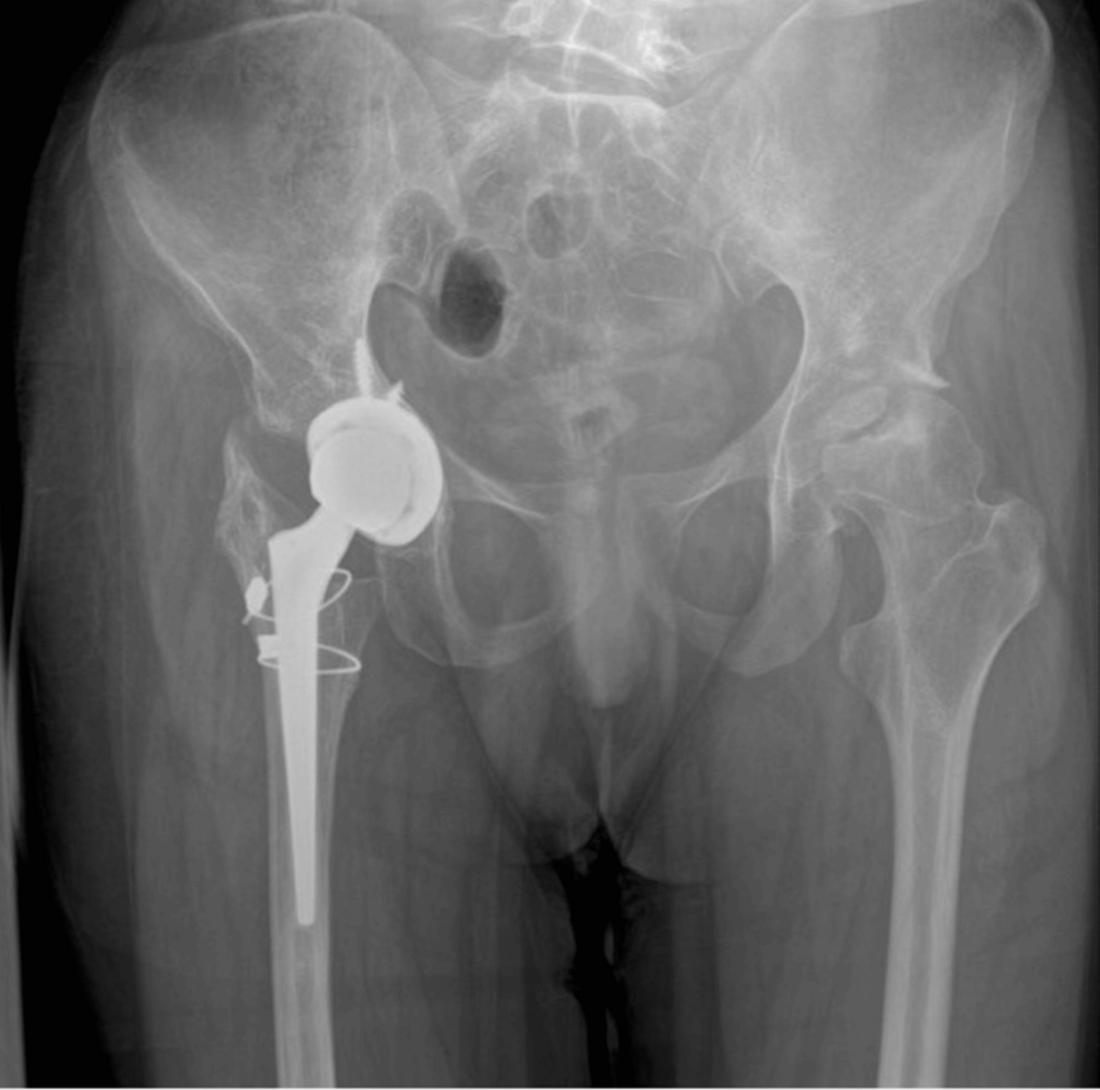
Immediate post-operative X-ray of the pelvis
